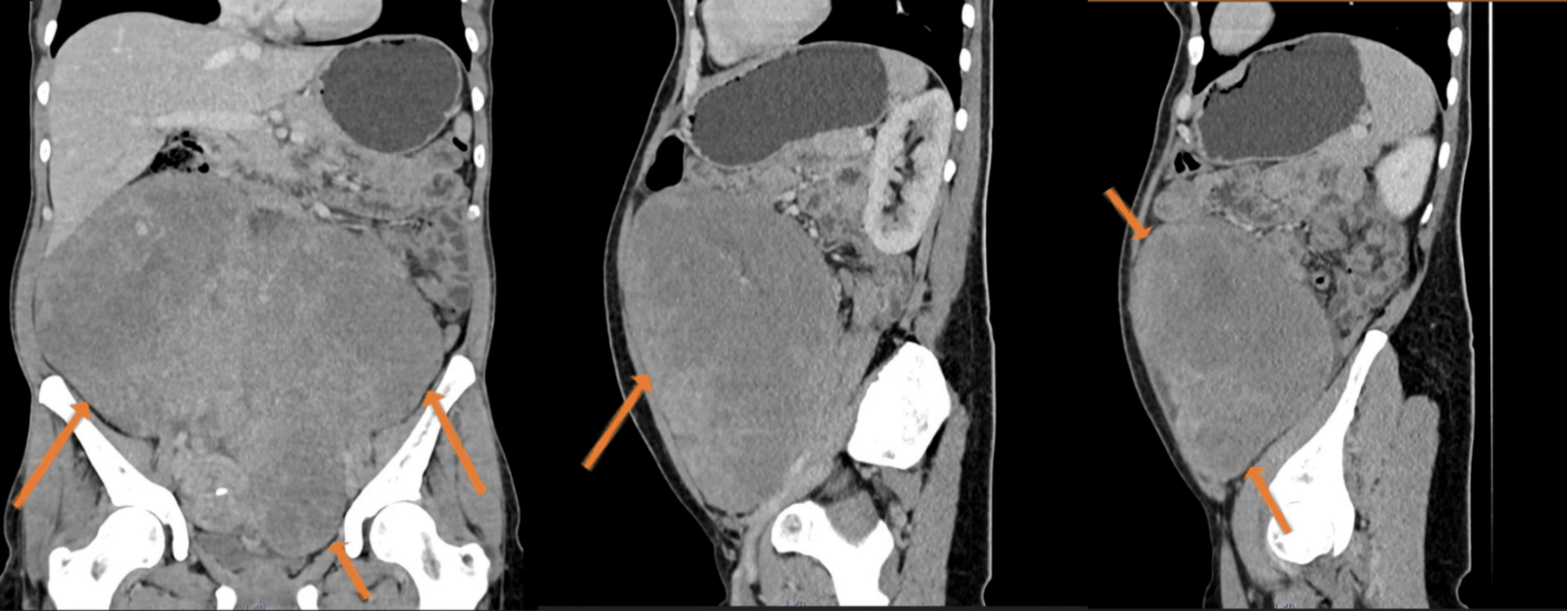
Images of an abdominopelvic CT scan that shows a giant mass (limited by arrows), which measures 30 x 28 x 16 cm, in the abdominal and pelvic cavity.
